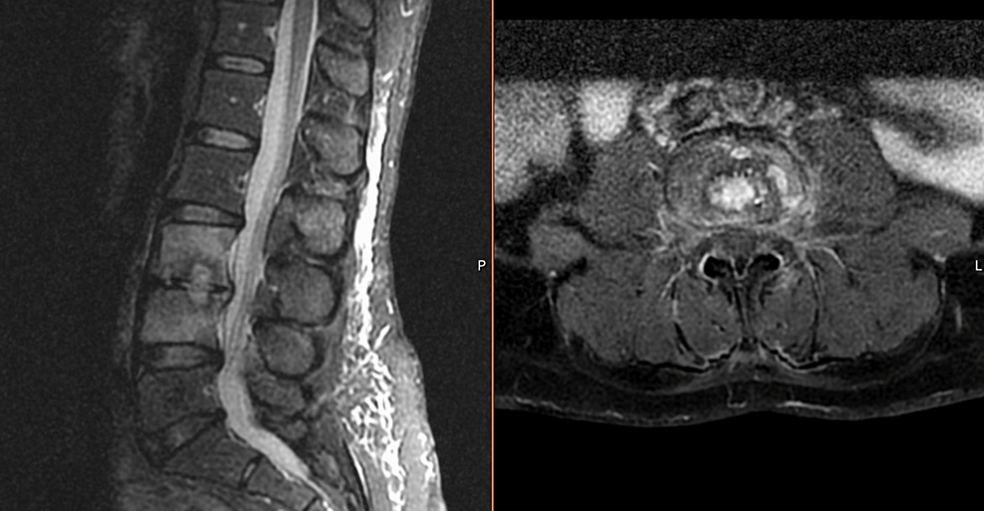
L3-L4 vertebral osteomyelitis with spondylodiscitis on magnetic resonance imaging.
